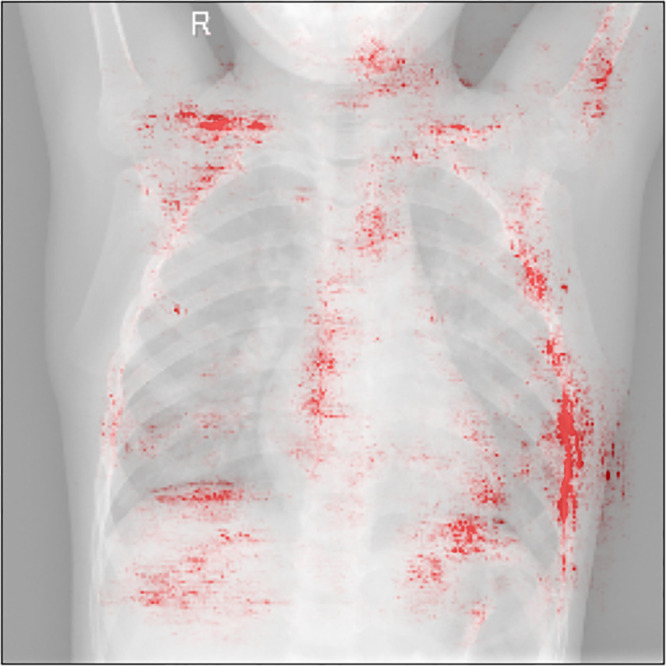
The attribution of each pixel of a radiograph in a healthy pediatric
